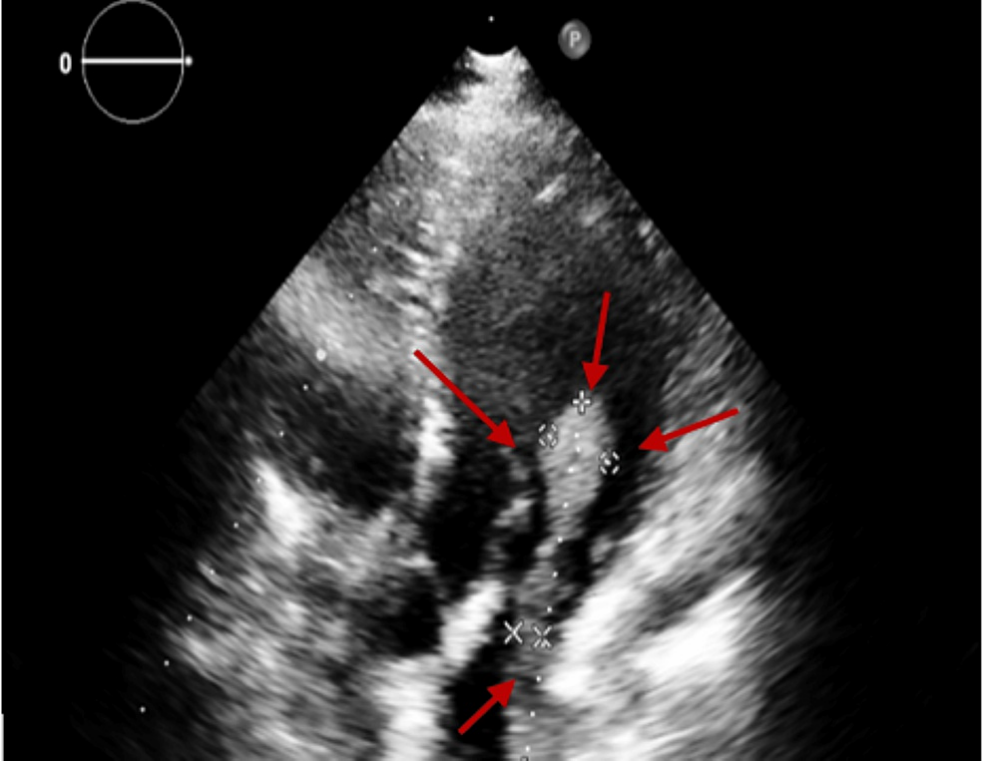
Transthoracic echocardiography modified apical five-chamber view showing an elongated mass (1.6 cm x 0.6 cm x 6.3 cm) protruding into the left ventricle during diastole
